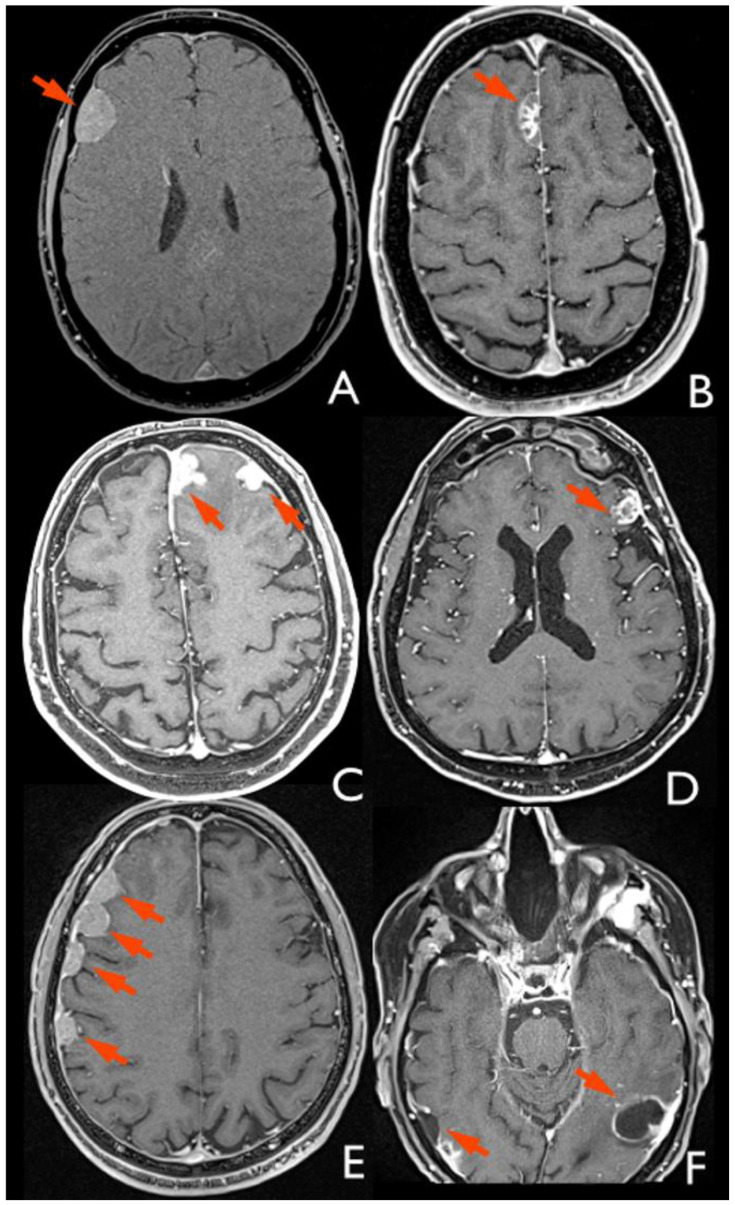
Images of dural implant metastases in MRI on axial plane with VIBE sequences. (A) Single metastasis in frontal location; (B) single metastasis of the anterior falx; (C) dual metastasis in frontal and anterior falx locations; (D) single metastasis of the left supraorbital gyrus; (E) multiple metastases in fronto-parietal location, some of which are confluent; and (F) cystic metastases in right and left occipito-temporal locations.
